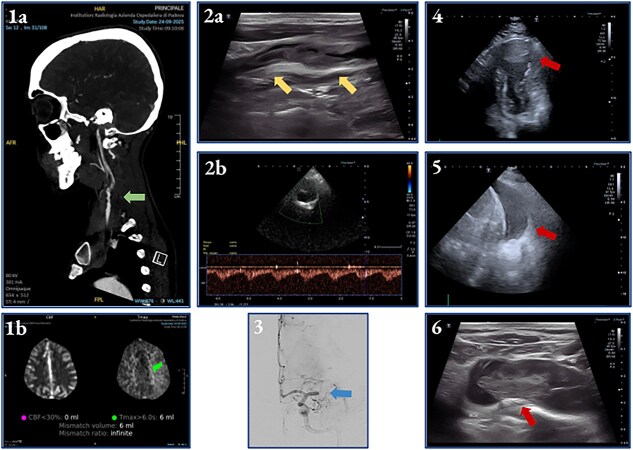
A 55-year-old woman with arterial hypertension and recent myocardial infarction woke up with acute right-sided weakness and facial drooping (NIHSS 4). Initial CT at 1 hour from stroke onset was normal, while CTA indicated distal common/internal carotid artery stenosis and a small hypoperfused area (1a–b). During IV thrombolysis, her neurological condition worsened (NIHSS 10). Brain-POCUS revealed a mobile thrombus in the left common/internal carotid artery with intracranial microembolic signals (2a–b) later confirmed by angiography showing carotid sub-occlusion and M1 segment occlusion (3). Endovascular thrombectomy achieved near-complete reperfusion (TICI 2c) , followed by carotid thromboaspiration and stenting. Cardio-POCUS, performed to investigate a possible cardiac embolic source, identified a left ventricular apical aneurysm with thrombus (4). Lung-POCUS performed for acute respiratory distress showed pleural effusion (5), while venous-POCUS detected subclavian vein thrombosis (6). High-resolution CT revealed lung cancer with pulmonary embolism, suggesting paraneoplastic hypercoagulability.
